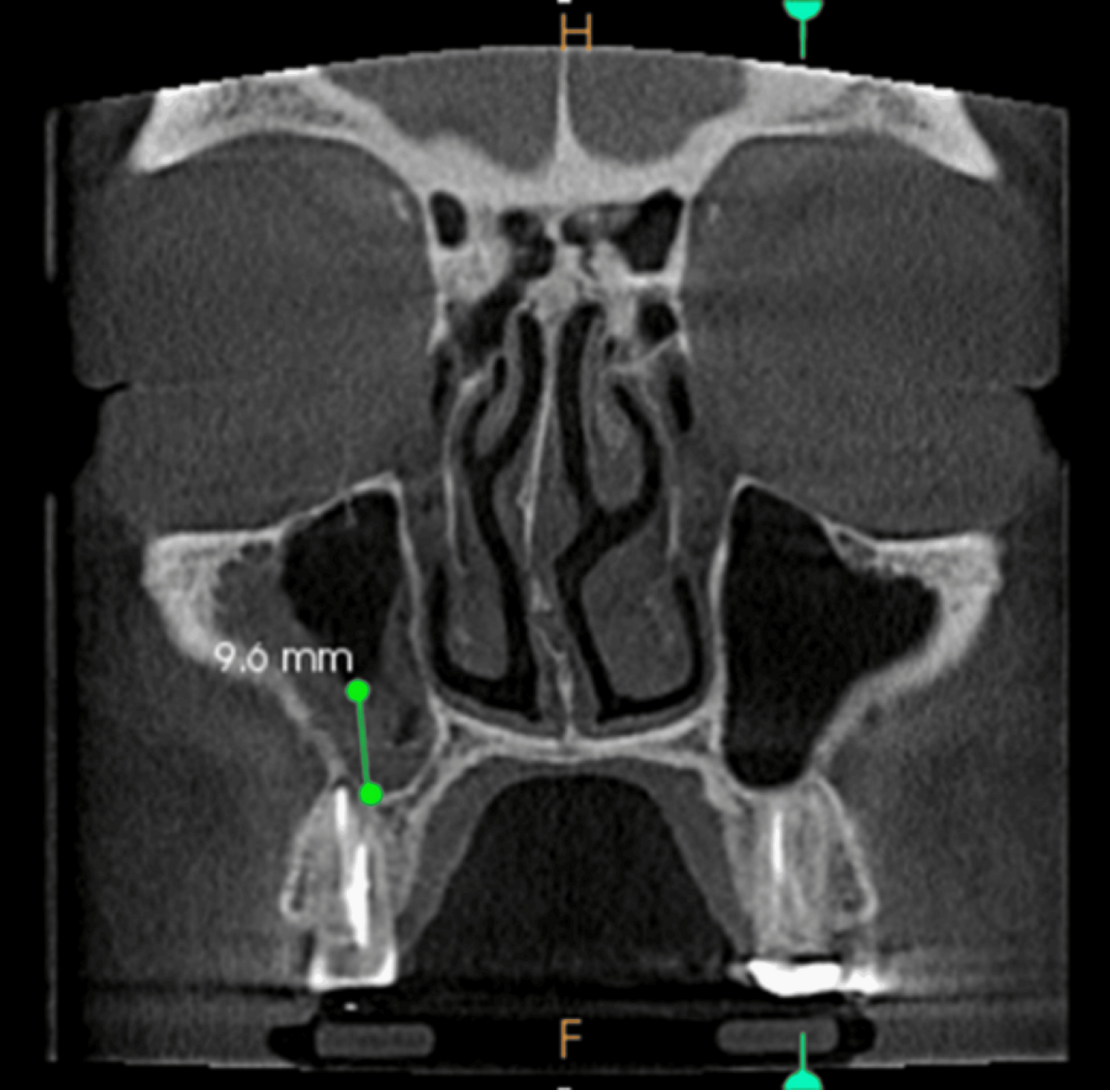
Periapical abscess in 15 with right maxillary sinus mucosal thickening
